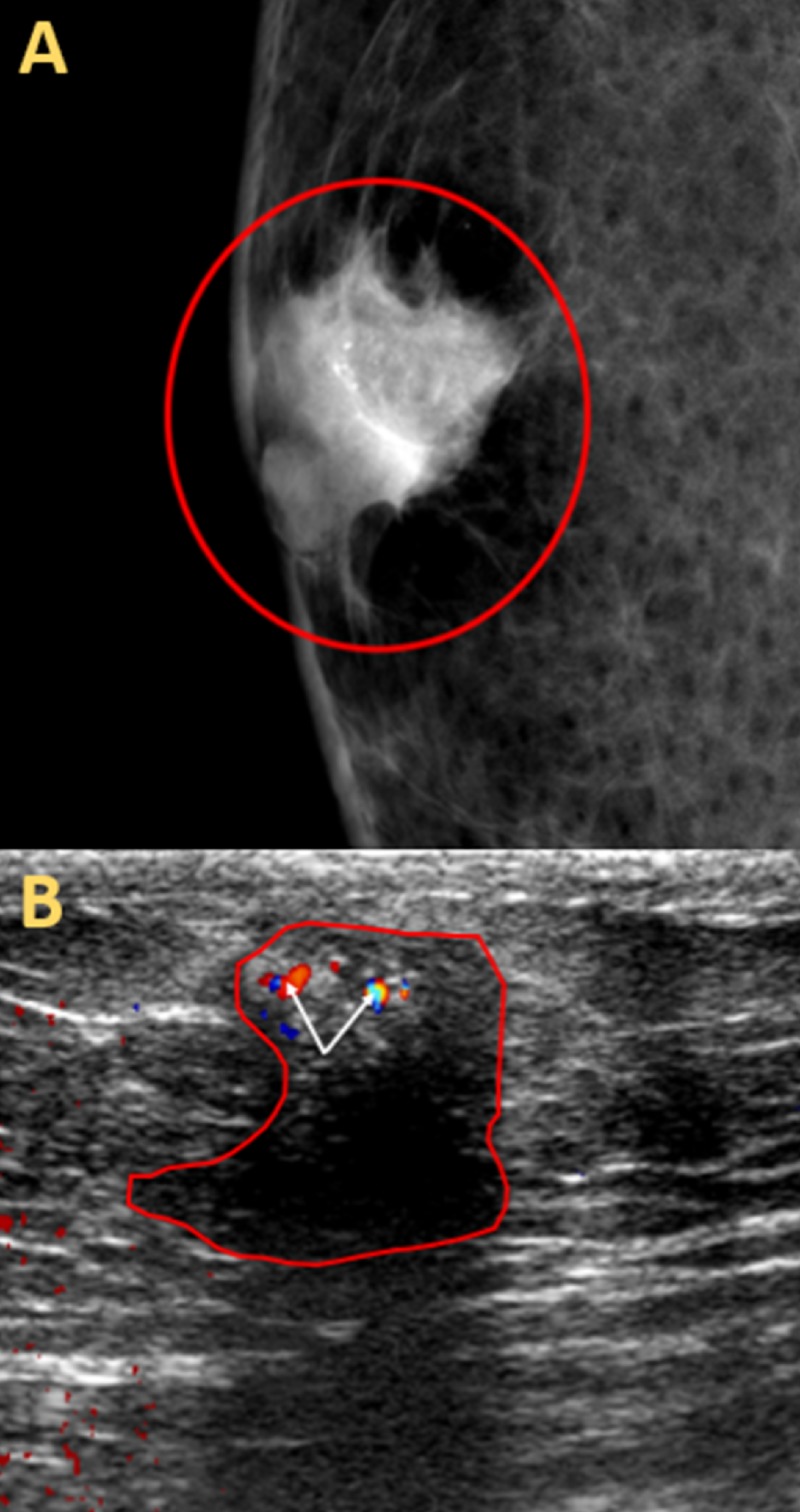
Imaging Findings of Infiltrative Ductal Carcinoma In Situ (A) A diagnostic mammogram of right breast shows an irregular mass with spiculated margins (red circle), measuring 15 millimeters with associated punctate calcifications in the subareolar region. (B) A diagnostic ultrasound of the right breast shows a hypoechoic irregular mass with angular margins (red outline) and internal vascularity (white arrows), measuring 15 millimeters in the subareolar region.
